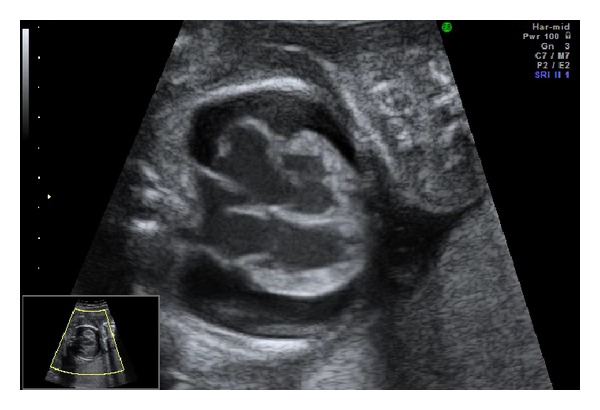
Fetal echocardiography at 21 weeks of gestation. A 2D image of the four-chamber view showed cardiomegaly, dilated ventricles, and atria with thickened and echo-dense walls, as well as massive pericardial effusion.
